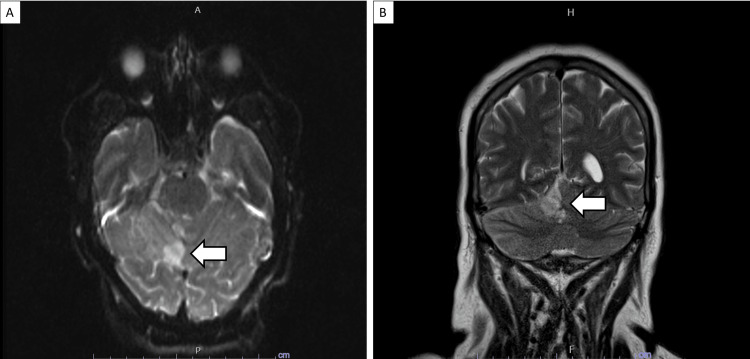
MRI of the brain showing right cerebellar stroke. MRI of the brain with and without gadolinium demonstrating right cerebellar infarction (white arrows) in the (A) axial DWI sequence and (B) coronal T2-weighted sequence. MRI, magnetic resonance imaging; DWI, diffusion-weighted imaging
